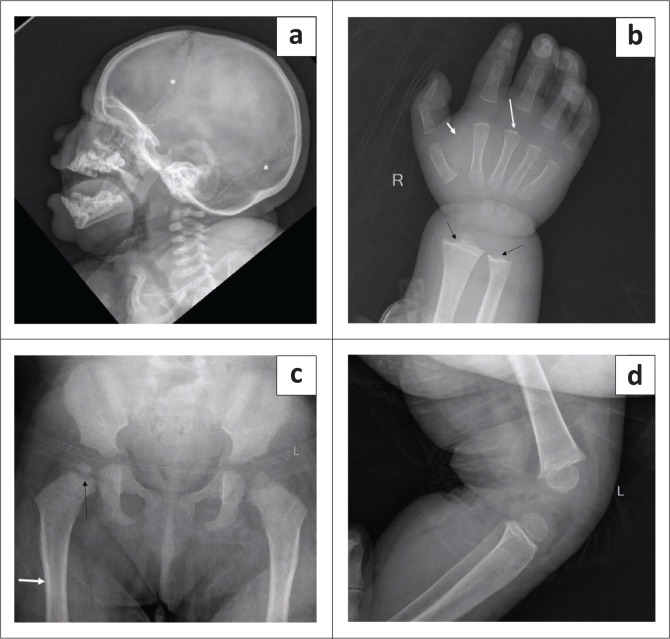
Lateral radiograph of the skull (a) showing multiple Wormian bones in the coronal and lambdoid sutures (*). Frontal radiograph of the right wrist (b) showing a delayed appearance of ossification centres for the carpal bones. Bone age corresponded to more than 1 year and less than 4 years. Metacarpal epiphyseal ends show a small spike-like projection extending into the growth plate (long white arrow). Irregularity of the growth plate is seen along the distal end of the radius and ulna (black arrows) and there is soft tissue hypertrophy (small white arrow). (c) Frontal radiograph of the pelvis and proximal femurs shows fragmented epiphysis (black arrow) with cortical thickening of both proximal femoral diaphysis (arrow in c). (d) The lateral radiograph of the left knee is unremarkable.
